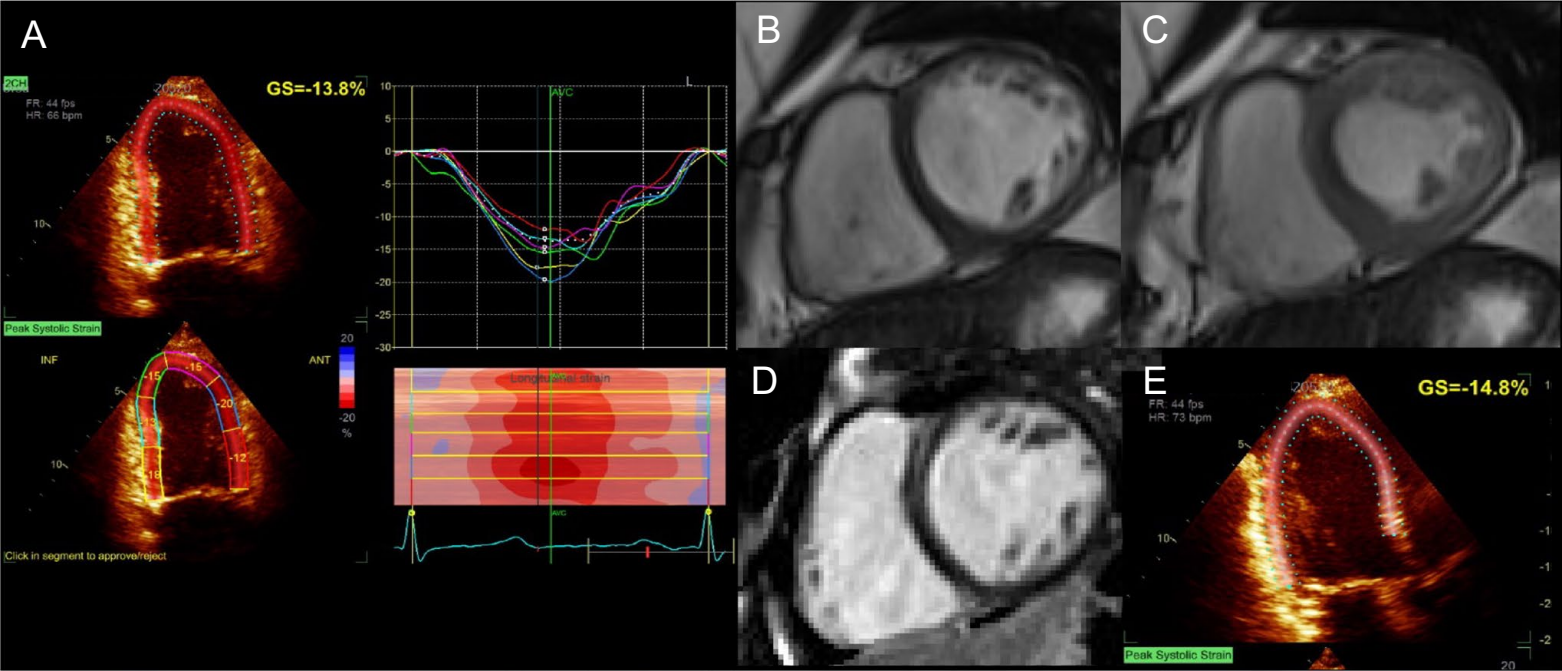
Acute onset of dyspnea in a 47-year-old woman with breast cancer. A 74-year-old woman without CVRFs presented with a human epidermal growth factor receptor 2 (HER2)-positive, hormone receptor (HR)-negative, locally advanced left-sided breast cancer with ipsilateral axillary lymph node extension (pT2N1M0, stage IIB). Given her age and the absence of indication for radiotherapy, she underwent surgical treatment by mastectomy and axillary lymph node dissection. Adjuvant chemotherapy was planned, consisting of 4 cycles doxorubicin (60 mg/m^2^) plus cyclophosphamide (500 mg/m^2^) and 12 cycles of weekly paclitaxel (75 mg/m^2^) plus trastuzumab (2 mg/kg) followed by 1 year of trastuzumab maintenance (3-weekly, 6 mg/kg). Pre-treatment echocardiography showed a normal LVEF (65%) and a normal GLS (18%). Following the third cycle [cumulative dose of anthracycline 180 mg/m^2^ (recommended maximum cumulative dose 400 mg/m^2^)], the patient presented with an acute onset of dyspnea requiring oxygen therapy. NT-proBNP and troponin I were increased (1026 ng/mL, ULN 738 ng/mL and 0.26 μg/L, ULN 0.10 μg/L, respectively). Echocardiography showed decreased LVEF (45%) and GLS (13.8%, N > 15%). A Doxorubicin-induced CTRCD was suspected, and a CMR was performed confirming reduced LVEF (42%). B and C Balanced SSFP short-axis view of mid-LV. LGE sequences showed no sign of scar (D). Chemotherapy was withheld and HF treatments consisting of diuretic, betablockers, and angiotensin-converting enzyme inhibitor were initiated, and control echocardiography showed an increase of LVEF but persistence of mildly decreased GLS (E), indicating persistent subclinical cardiotoxicity. Any further exposure to doxorubicin was omitted. Continuation of the treatment with paclitaxel and trastuzumab was planned to be initiated when LVEF would recover to approximately 50% under regular echocardiographic and laboratory monitoring. With breast cancer becoming the most prevalent cancer worldwide in 2020 [234], a shift of the overall burden of cancer treatment-related cardiac complications towards women can be expected. Increasing awareness about the intertwining of cardiac diseases and cancer is therefore paramount to cardiac imagers. The detection of subclinical cardiotoxicity before the onset of heart failure using advanced imaging criteria, such as GLS, or obtaining cardiovascular information from oncological imaging exams [213] could help to reduce the complications of cancer treatments and should therefore be part of the routine monitoring of patients undergoing potentially cardiotoxic therapies. Abbreviations: *CMR*: cardiac magnetic resonance;* CTRCD*: cancer treatment-related cardiac disease; *CVRF*: cardiovascular risk factor; *GLS*: global longitudinal strain; *HF*: heart failure; *LGE*: late gadolinium enhancement; *LV*: left ventricle; *LVEF*: left ventricular ejection fraction; *NT-proBNP*: N-terminal brain natriuretic peptide; *SSFP*: steady-state free precession; *ULN*: upper limit of normal
